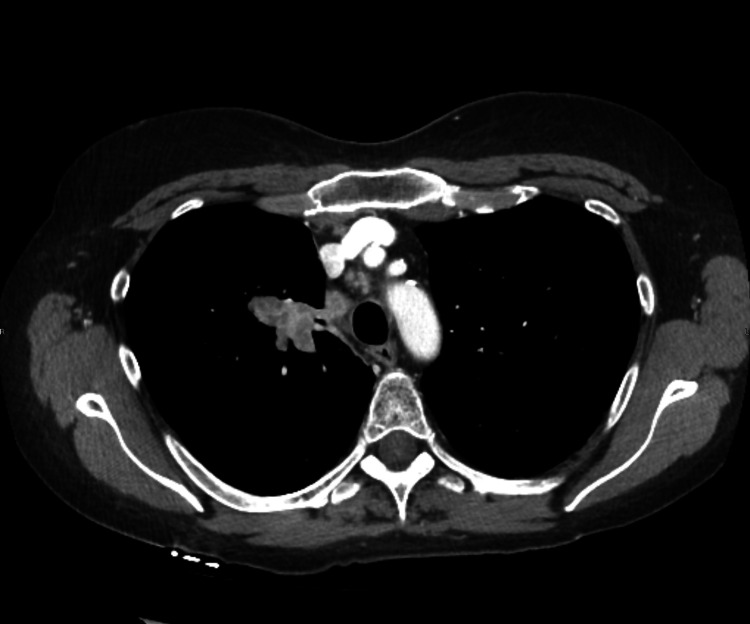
Contrast CT scan of the chest. Contrast CT of the chest revealed a 5.8 cm x 3.8 cm x 2 cm spiculated right upper lobe mass. The mass abuts the right upper lobe bronchus with narrowing of a posterior segmental bronchus.
